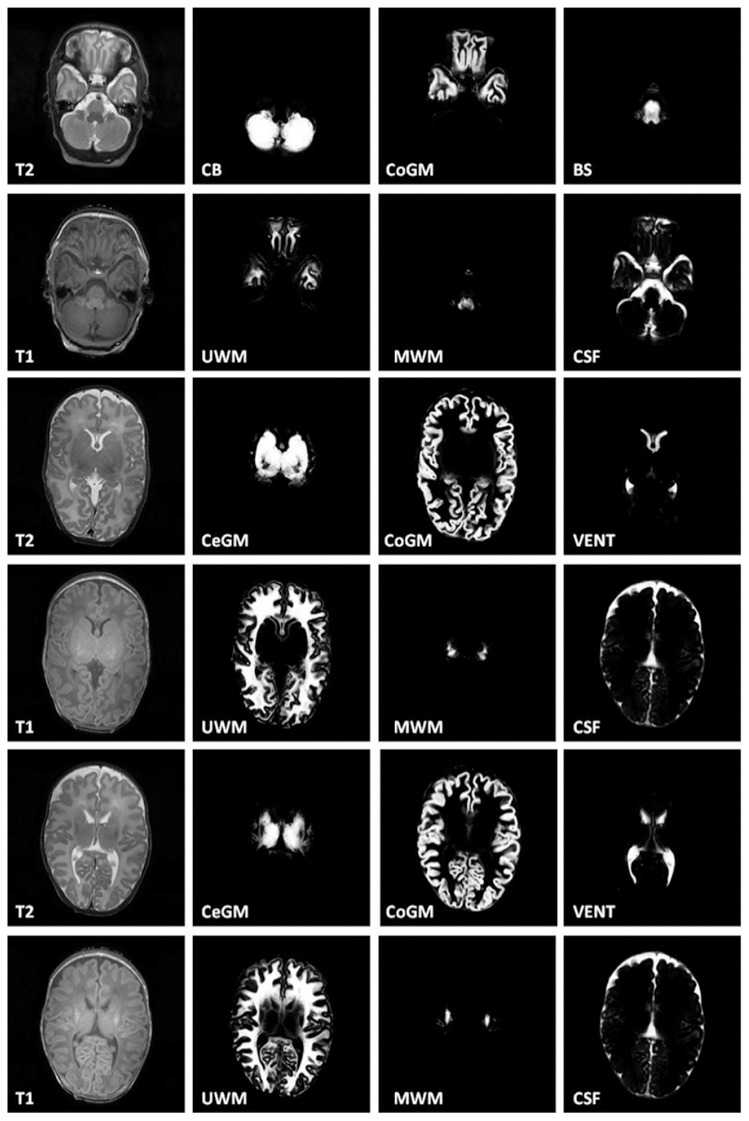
Probabilistic segmentations of intracranial tissues on several levels. Abbreviations: T2: T2-weighted image; CB: Cerebellum; CoGM: Cortical gray matter; BS: Brainstem; T1: T1-weighted image; UWM: Unmyelinated white matter; MWM: myelinated white matter; CSF: Cerebrospinal fluid.
